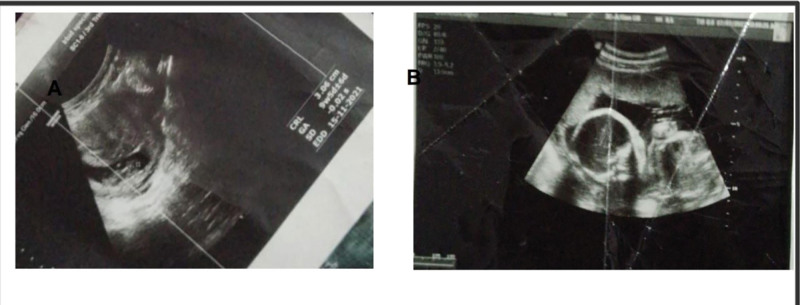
Prenatal ultrasound at 31 wk gestation. (A) Longitudinal fetal lie with unstable positioning. (B) Cross-sectional image showing transverse position. These findings contributed to the decision for cesarean delivery. Preterm birth, along with low birth weight (800 g), increases the risk of neurodevelopmental delay and structural abnormalities, including ventriculomegaly and skeletal anomalies.^[8–10]^
